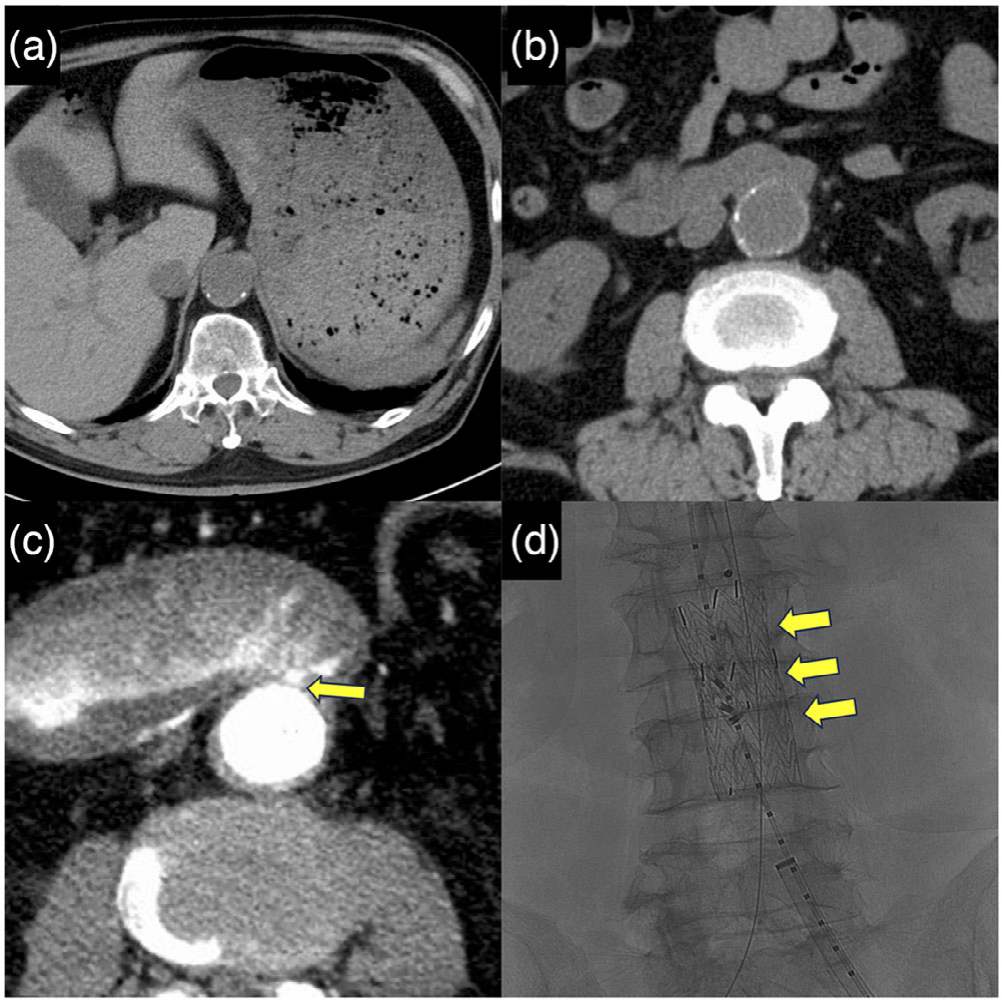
Computed tomography (CT) findings. (a) Non‐contrast CT revealing high‐attenuation material in the stomach, measuring approximately 40–50 Hounsfield units, suggestive of massive gastrointestinal bleeding. (b) Close anatomical proximity between the aorta and the horizontal portion of the duodenum; however, no aortic aneurysm or intraluminal air is detected. (c) Contrast‐enhanced CT demonstrating extravasation of contrast medium from the aorta into the horizontal portion of the duodenum, raising suspicion for secondary aortoduodenal fistula (arrow). (d) Emergency endovascular aortic repair (EVAR) resulted in hemostasis (arrow).
